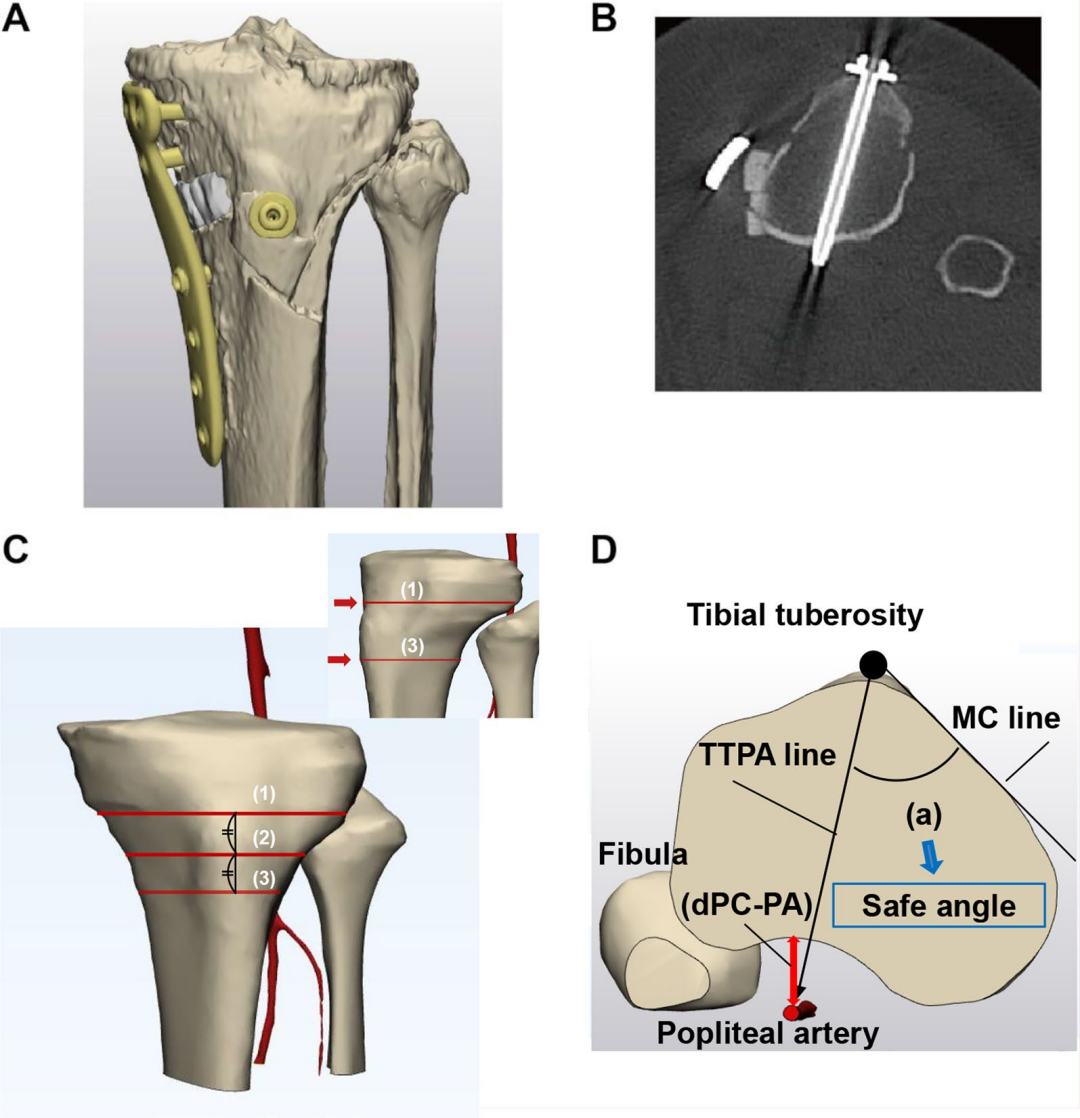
A Three-dimensional bone models after DTO. B An axial plane in the CT image after DTO: an anteroposterior screw for bicortical fixation of the tibial tuberosity in DTO. C Most proximal point (1), midpoint (2), and most distal point (3) in the tibial tuberosity. D An axial plane. (a): the angle between medial cortex (MC) line and tibial tuberosity and popliteal artery (TTPA) line. dPC-PA: the distance between the posterior cortex and the popliteal artery
